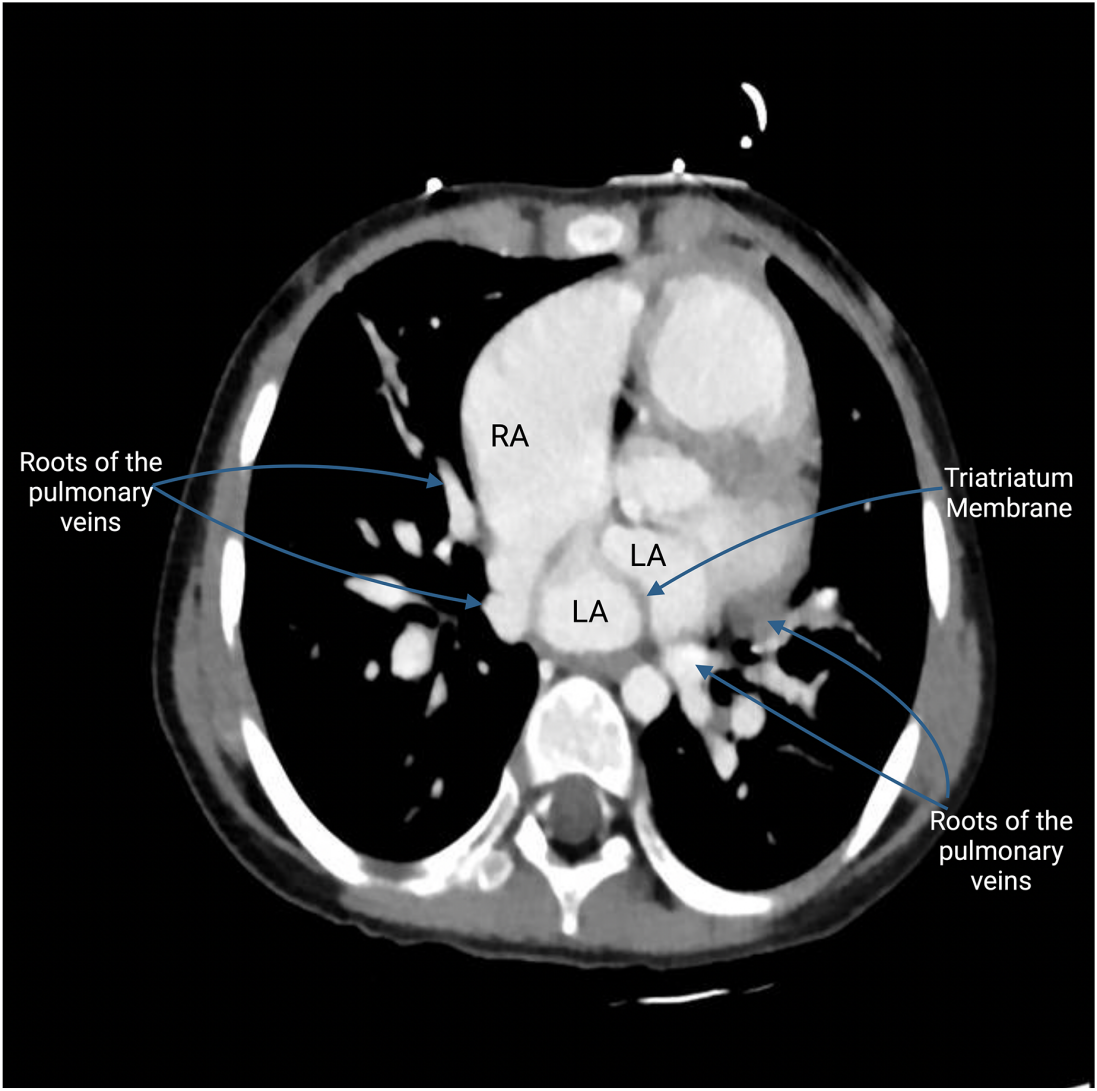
This CT image highlights the fibromuscular membrane dividing the left atrium into two chambers. Visible on the scan is the Cor membrane, which acts as a partition within the atrium, creating a distinct proximal and distal chamber. The proximal chamber receives the pulmonary veins, while the distal chamber channels blood to the mitral valve, leading to the left ventricle. The scan shows the unusual entry points of the pulmonary veins: the roots of two pulmonary veins are depicted entering the roof of the right atrium, an anomalous connection indicative of PAPVR. In contrast, the roots of the two other pulmonary veins are seen entering the left atrium, following the normal anatomical pattern. This difference between normal and abnormal venous connections highlights the unique challenges posed by Cor Triatriatum.
